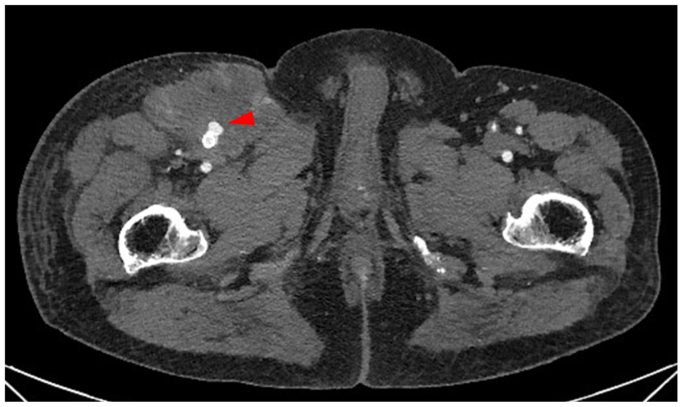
Computed tomography (CT) angiography revealing a pseudoaneurysm (*arrow*) at the proximal segment of the right superficial femoral artery (SFA).
